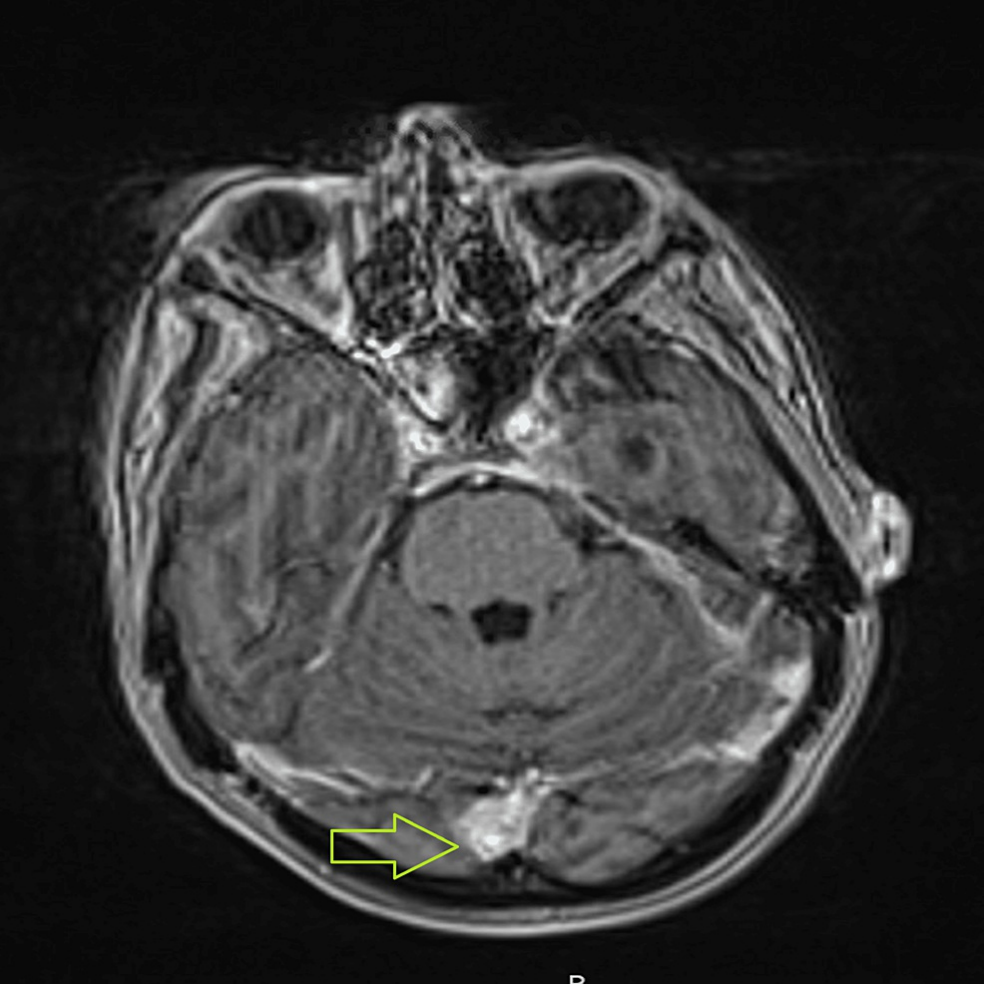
Three months post-CAR-T MRI of the brain revealed enhancement affecting left-sided Meckel's cave, cisternal trigeminal nerve, and left internal auditory canal, with increased prominence of enhancement into the left inner ear structures and along the left seventh nerve
